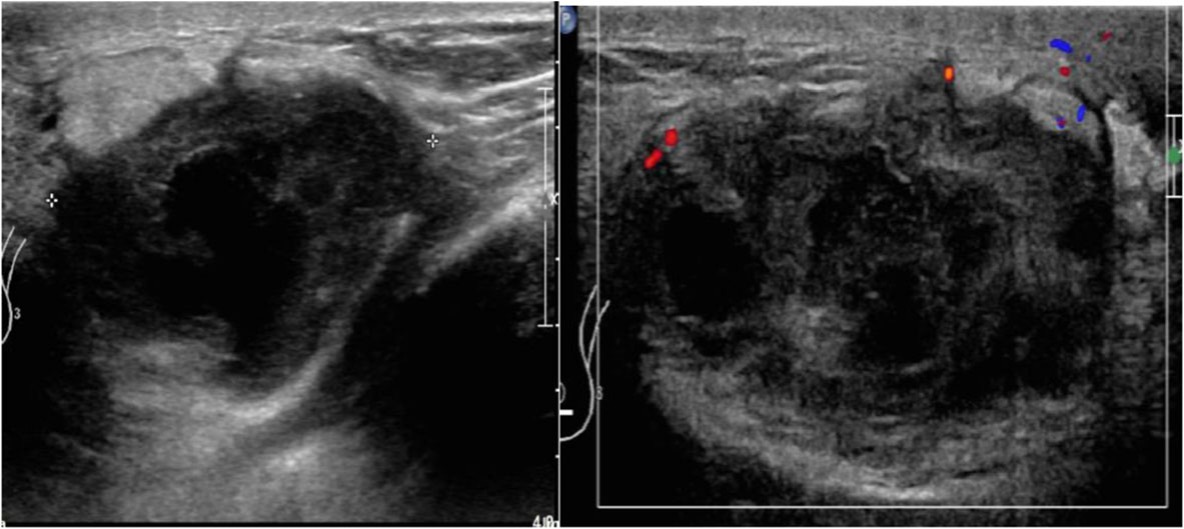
Ultrasound of the left breast
